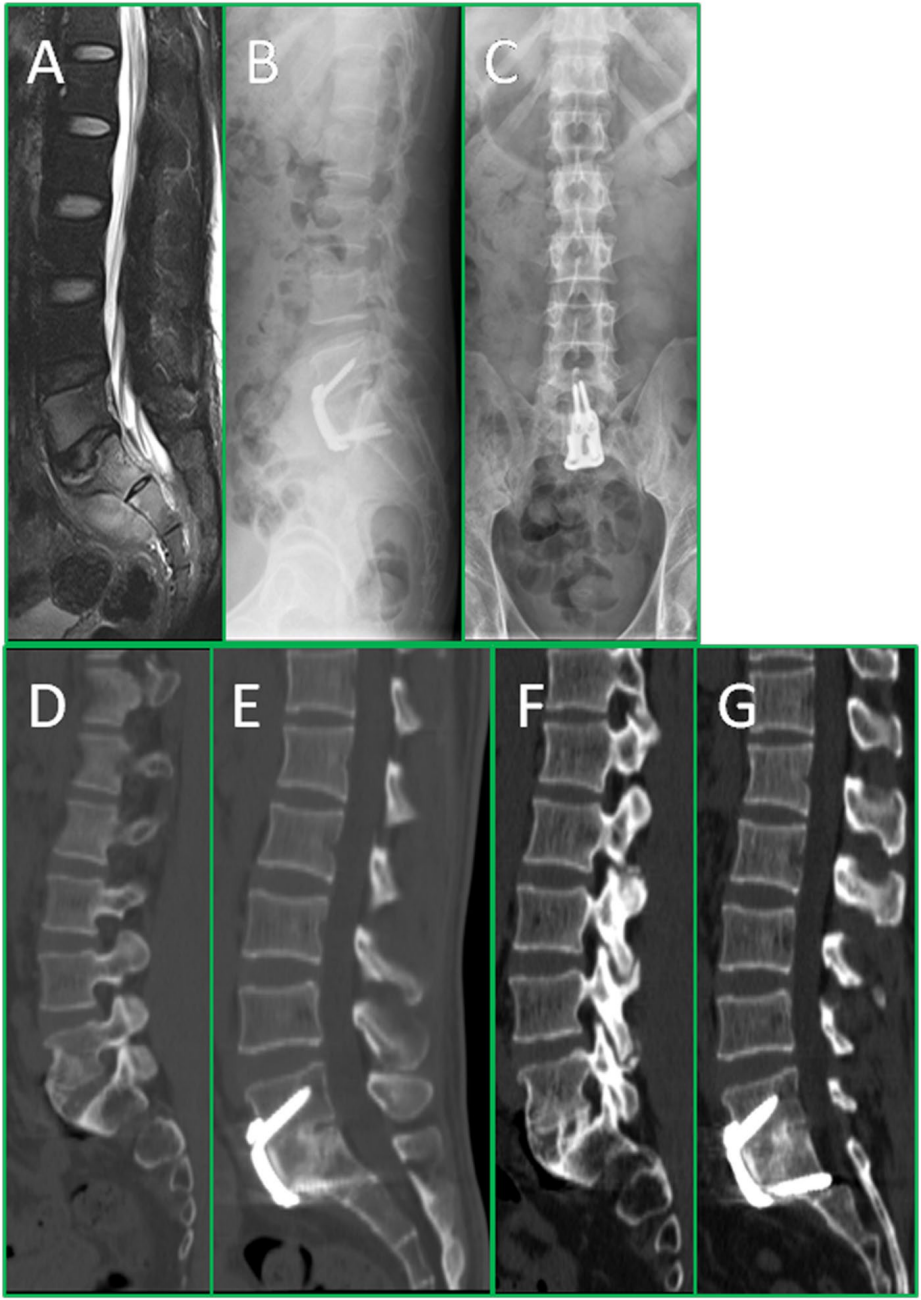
The series of radiographs show the radiological changes before and after treatment. This is a 35-year-old male patient, who had L5-S1 spinal TB with pre-sacral abscess, low back pain, and right lower limb radicular pain. The pre-operation MRI (A) shows bone destruction of L5 and S1 and pre-sacral cold abscess formation; The post-operative X-ray (B,C) confirmed the position of plate; The 12 months post-operative X-ray (D,E) shows definite bone fusion, while intervertebral height and the lumbosacral angle maintained satisfactory correction; The 24 months post-operative X-ray (F,G) reveals the ARCH plate still in position and good inter-body fusion of L5-S1, and the intervertebral height and lumbosacral angle were well maintained.
